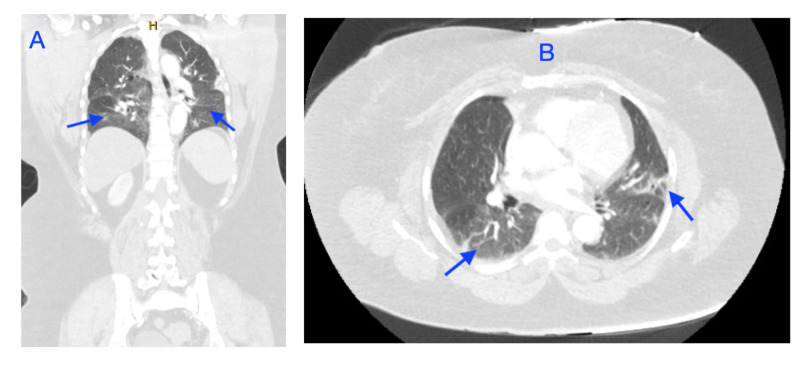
Computer tomography of the chest, abdomen and pelvis with IV contrast CT chest transversal cut showing bilateral ground-glass opacities (A) and CT chest coronal cut showing bilateral ground-glass opacities (B).
